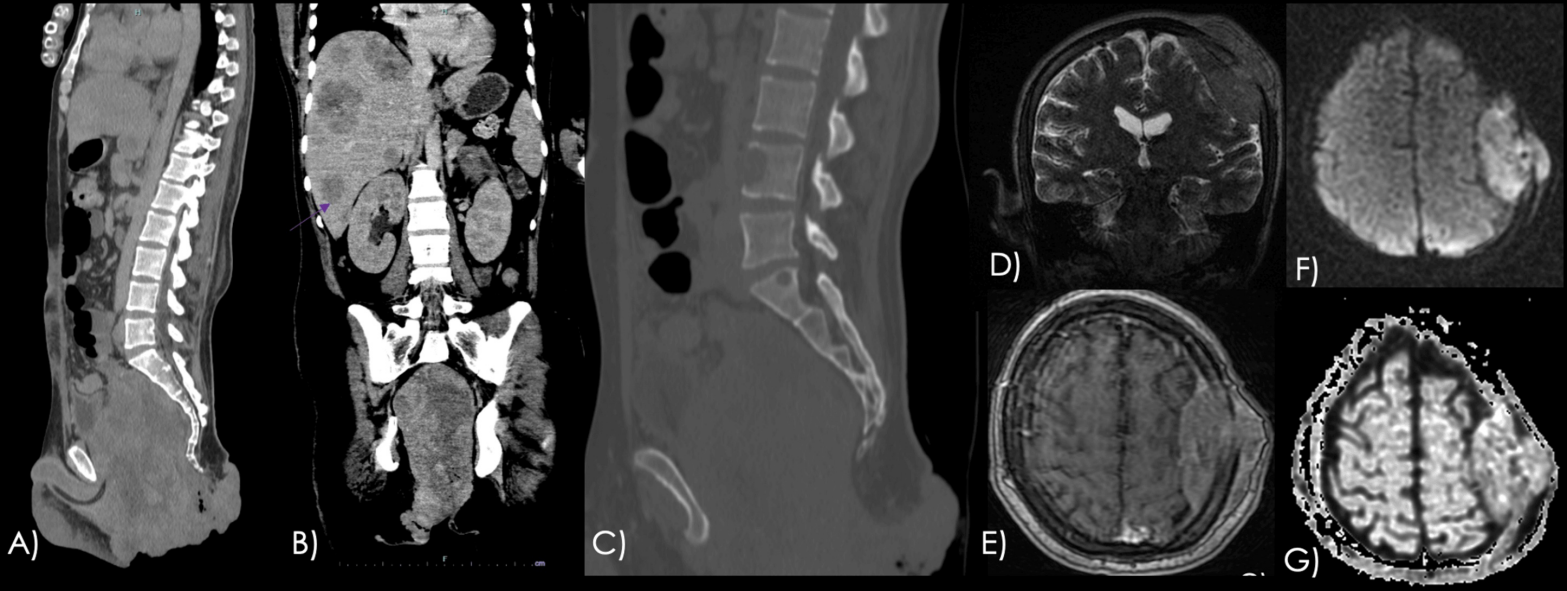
Follow-up imaging showing recurrence and disease progression Contrast-enhanced CT abdominal scan. A large, irregular, and infiltrative mass is identified, involving the entire pelvic cavity, infiltrating the corpora cavernosa, and displacing the bladder (A). Multiple rounded lesions with diffuse distribution and heterogeneous appearance are seen, consistent with metastatic disease (B). In the bone window, lytic lesions are identified in multiple lumbar vertebral bodies (C). Contrast-enhanced brain MRI T2-weighted coronal (D), T1-weighted axial post-gadolinium (E), axial DWI (F), and ADC map (G) images show an extra-axial supratentorial lesion in the left frontoparietal region, demonstrating homogeneous contrast enhancement. The lesion involves the calvarium, originates from the meninges, and shows marked diffusion restriction. The corresponding ADC value was measured at 0.67 × 10^-3^ mm^2^/s, consistent with true diffusion restriction, in keeping with lymphoma. CT, computed tomography; MRI, magnetic resonance imaging; DWI, diffusion-weighted imaging; ADC, apparent diffusion coefficient
